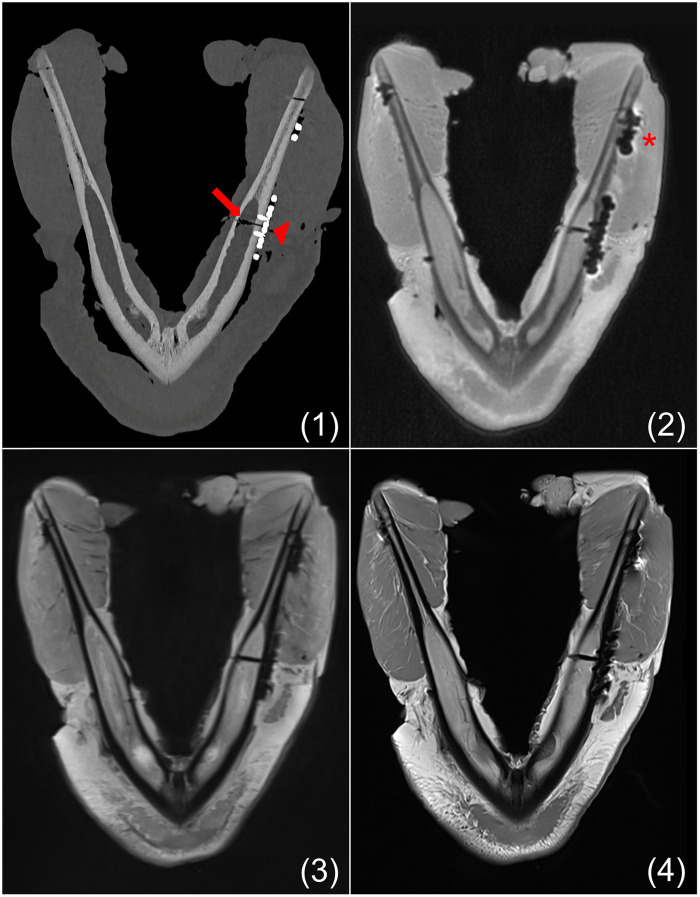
CT versus metal artifact-reduced MRI. Axial imaging of the mandible after osteosynthesis: CT (1) vs. MRI (2–4) with advanced metal artifact-reduction sequences. From left to right and from top to bottom: (1) Photon-counting CT, (2) UTE, (3) SEMAC and (4) WARP. A body fracture is depicted on the left side of the mandible (red arrow in 1) with an adjacent plate osteosynthesis (red arrowhead in 1). Osteosynthesis material and corresponding metallic artifacts are also visible at the mandibular angle (red asterisk in 2). CT, computed tomography; MRI, magnetic resonance imaging; UTE, ultrashort echo time; SEMAC, slice encoding for metal artifact correction; WARP, vendor-specific artifact reduction techniques (Siemens Healthineers, Forchheim, Germany).
